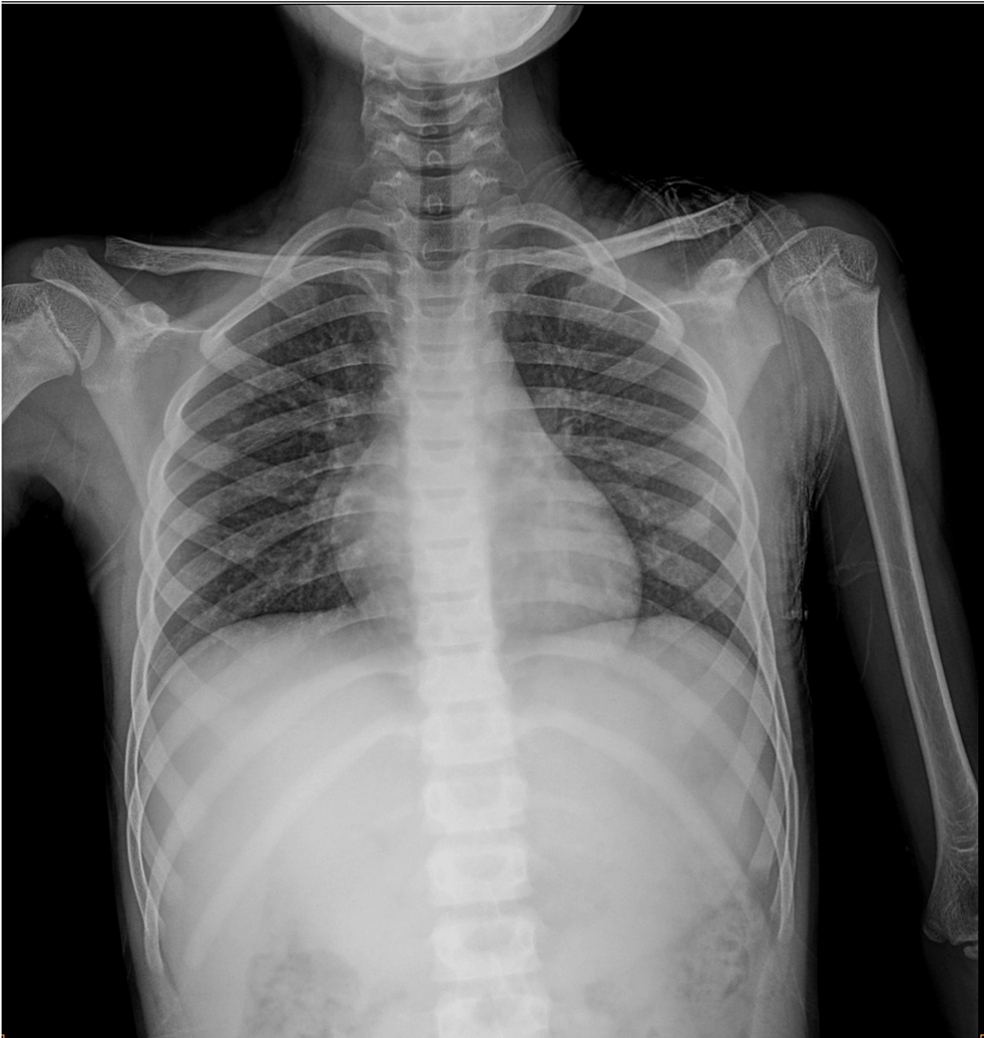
Post-extraction chest X-ray without abnormalities.
